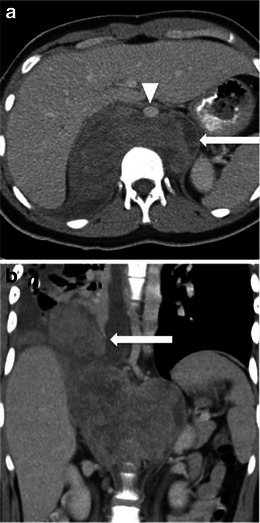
Imaging of synovial sarcoma. An 18-year-old man who presented with shortness of breath. a Axial contrast-enhanced CT shows a large heterogeneous retroperitoneal/retrocrural soft-tissue mass (*arrow*), which is displacing the aorta (*arrowhead*). b Coronal CT shows the extension of the retroperitoneal mass into the mediastinum. There is a right-sided pleural effusion and soft tissue pleural metastases (*arrow*)
